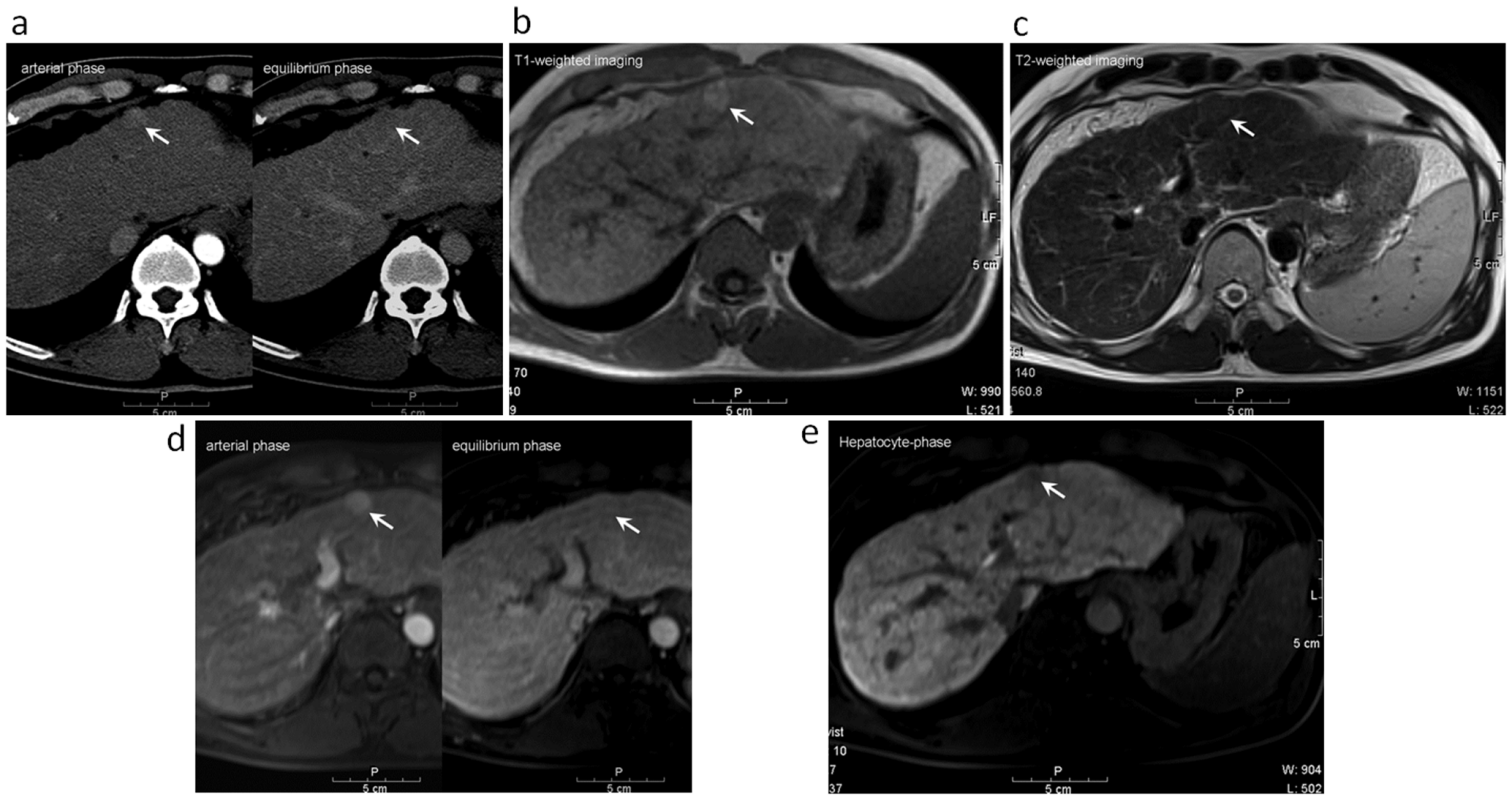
A 59-year-old male with a well-differentiated hepatocellular carcinoma (indicated by arrow) at S3 of the liver underwent gadoxetic acid-enhanced MR imaging and liver biopsy. (a) The tumor depicted hypervascular enhancement in the arterial-phase and isodensity relative to the adjacent liver parenchyma on the equilibrium phase of dynamic CT study. (b) The tumor showed hyperintensity on the T1-weighted image. (c) The tumor showed isointensity on the T2-weighted image. (d) The tumor also showed hypervascular enhancement in the arterial phase image and isointensity relative to adjacent liver parenchyma on equilibrium phases of the dynamic MR study. (e) The tumor showed hypointensity on the gadoxetic acid-enhanced hepatocyte-phase T1-weight image.
